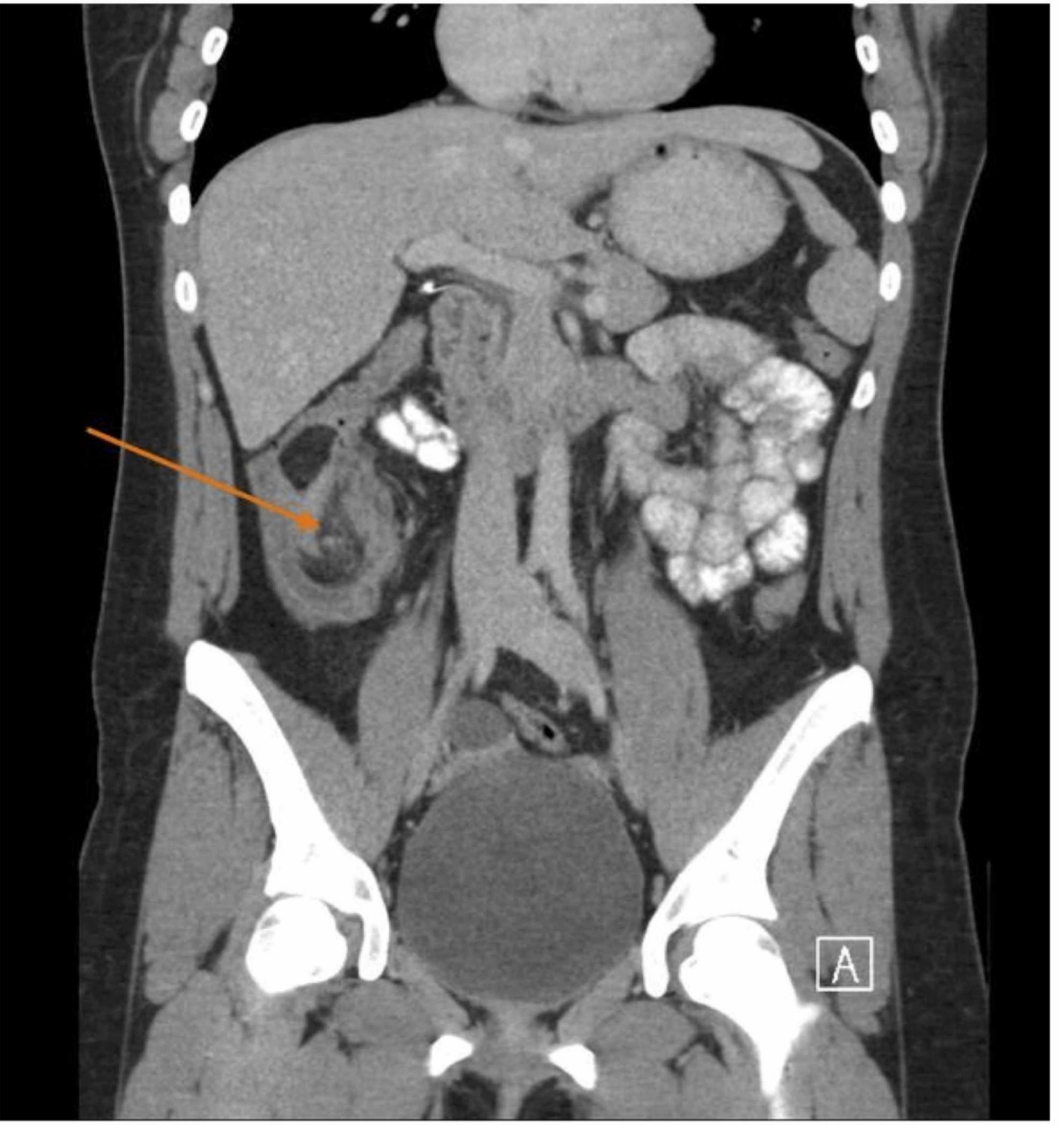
Computed tomography of abdomen and pelvis with contrast The axial view of a computed tomography scan of abdomen and pelvis with contrast. An ileocolic intussusception is present (orange arrow) with the terminal ileum telescoping into the ascending colon over an 8-cm interval.
